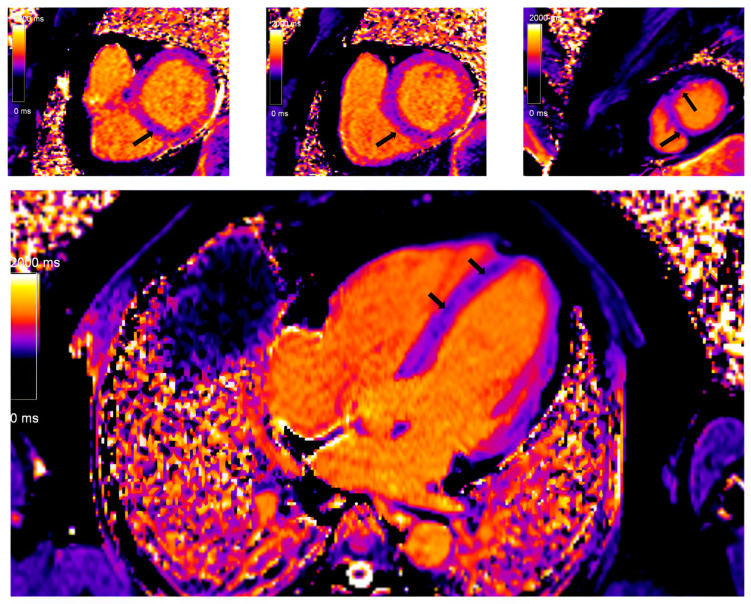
Myocardial tissue mapping in a patient with cardiac sarcoidosis and high degree AV block: native T1 mapping revealing the areas of shortened native T1 time indicating myocardial fibrosis predominantly in the septum (marked with arrows) (Avanto MRI, Siemens Healthcare GmbH, Erlangen, Germany, 1.5 T, CMR Lab University Clinical Hospital Center Bezanijska kosa, Belgrade, Serbia).
